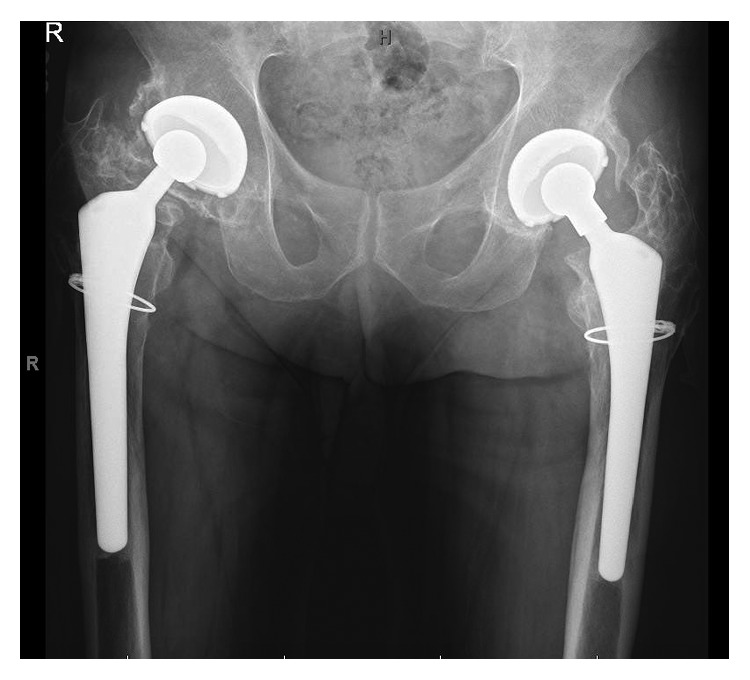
Plain radiograph of pelvis in a patient with history of bilateral periprosthetic infections 20 years following bilateral total hip arthroplasties. X-ray demonstrates good fixation of implant without loosening, some eccentric wear on the right polyethylene liner, no periosteal reaction. There is bilateral Brooker III/IV heterotopic ossification.
